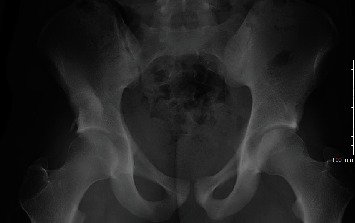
Postoperative X-ray 1 year after heterotopic bone removal.
